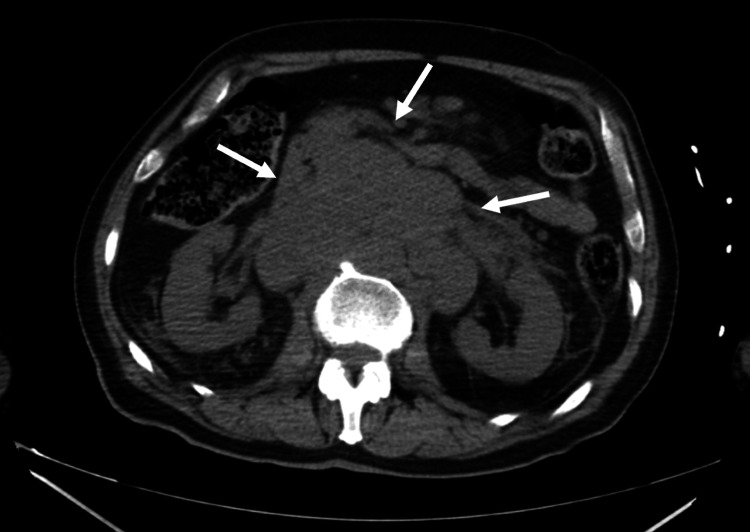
Axial abdominal CT showing a retroperitoneal lymphadenopathy conglomerate measuring 12 x 6 cm (white arrows). CT, computed tomography.
